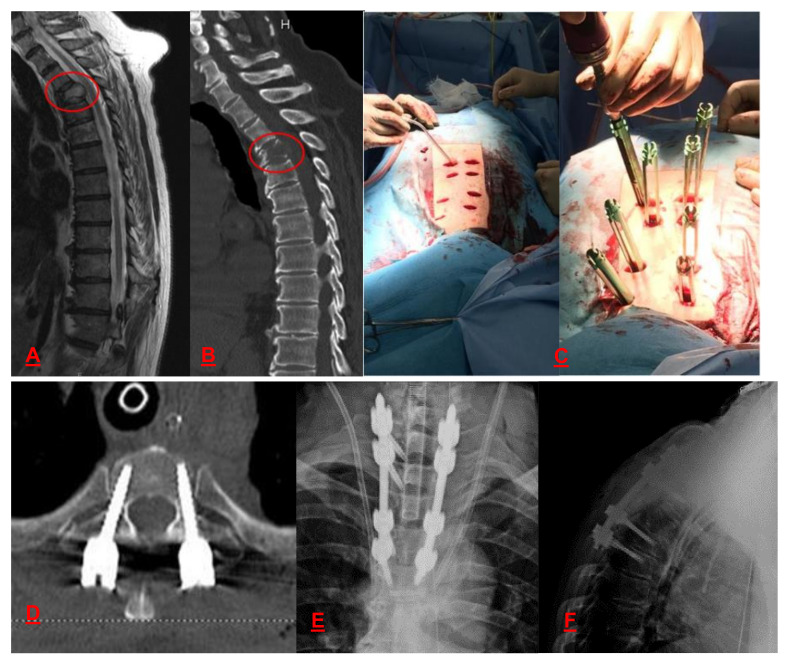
A 72-year-old man with T3 bone metastasis from prostate cancer treated with minimally invasive spine stabilization with percutaneous pedicle screws. (A) Sagittal T2-weighted magnetic resonance image shows a tumor bulging to the spinal canal. (B) Sagittal computed tomography (CT) shows a lytic lesion in the T3 vertebra. (C) Intra-operative photograph shows percutaneous pedicle screws osteosynthesis. Post-operative (D) axial, (E) anteroposterior, and (F) lateral CT radiographs show transpedicular screws osteosynthesis.
